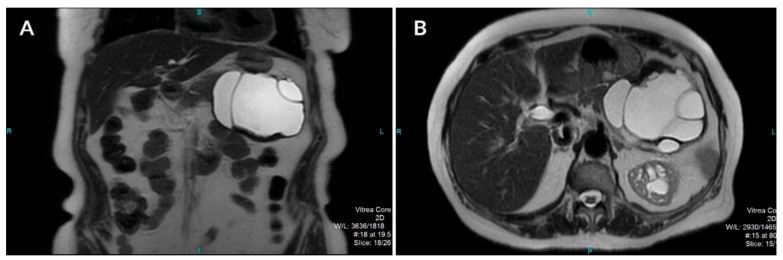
Coronal (A) and axial (B) T2-weighted images show a well-defined cystic lesion in the tail of the pancreas with high-signal-intensity inner loculi and a thick, complete hypointense rim with internal membranes.
